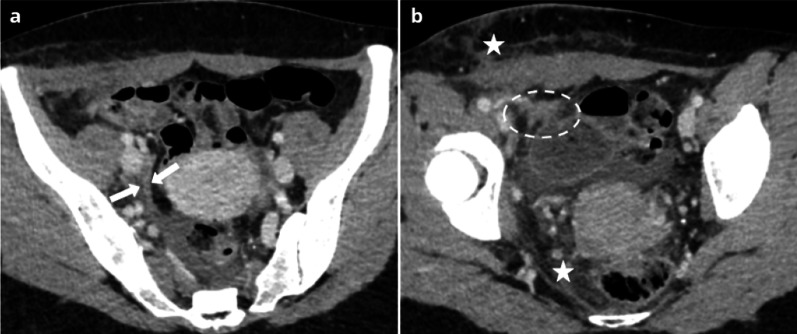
27-year-old female presenting with acute right lower quadrant pain and elevated CRP. CT (a, b) shows a normal appendix (arrows), but subcutaneous and pelvic fat stranding (star), along with a stellar retractile mass of the right iliac fossa (dotted circle). Surgical exploration found no sign of appendicitis or of right adnexal abnormality, but it revealed inflammatory peritoneal endometriotic implants of the right lower quadrant. A good clinical outcome was achieved with hormonal treatment
